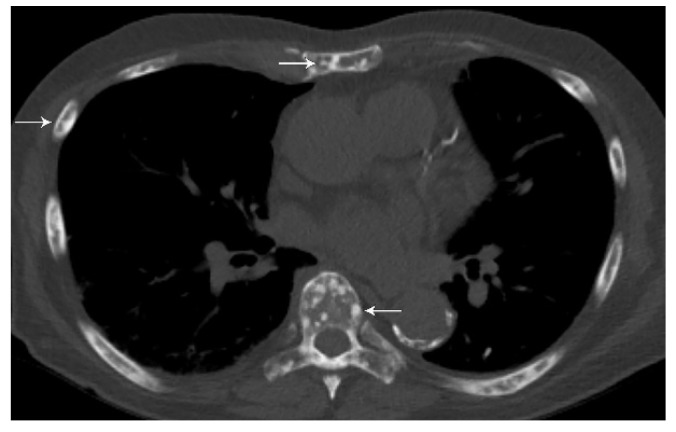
M1c disease, multiple extrathoracic metastases. CT shows multiple sclerotic bone metastases in the ribs, sternum, and spine (arrows). M1c disease, multiple extrathoracic metastases, constitutes stage IVB.
